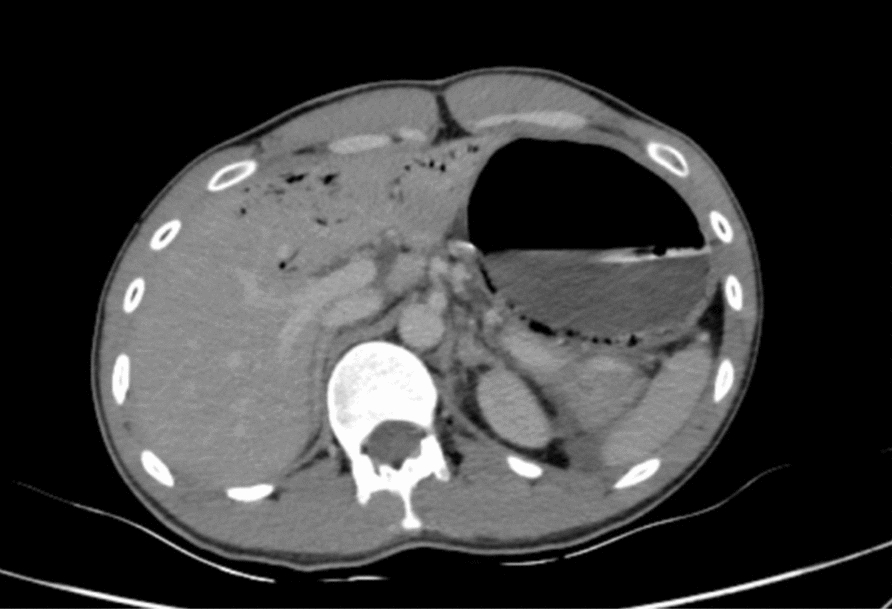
Axial computed tomography showing gastric emphysema and portal venous gas
